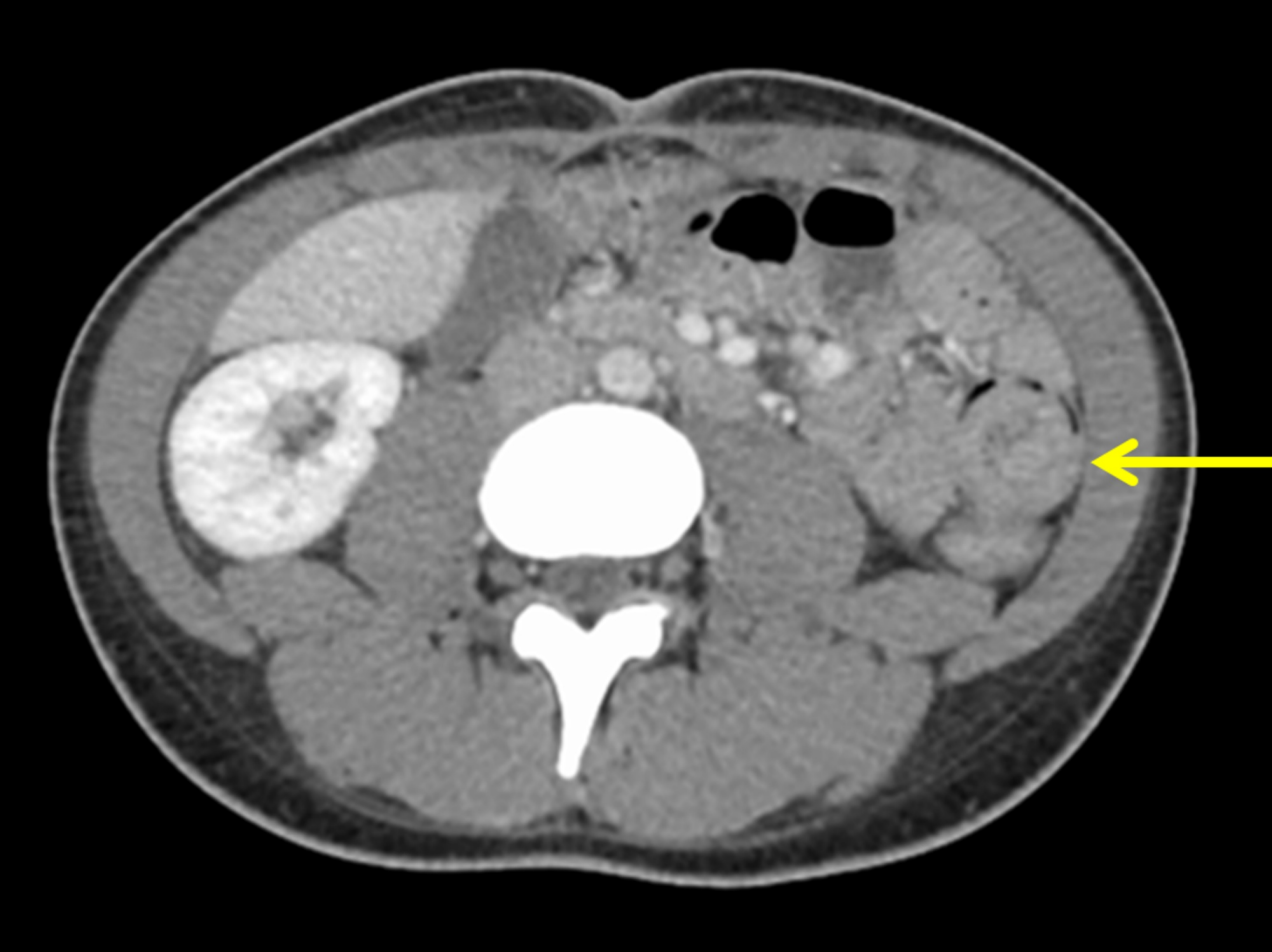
Computed tomography (CT) scan demonstrating intussusception in the left hemi-abdomen (yellow arrow) in our patient
